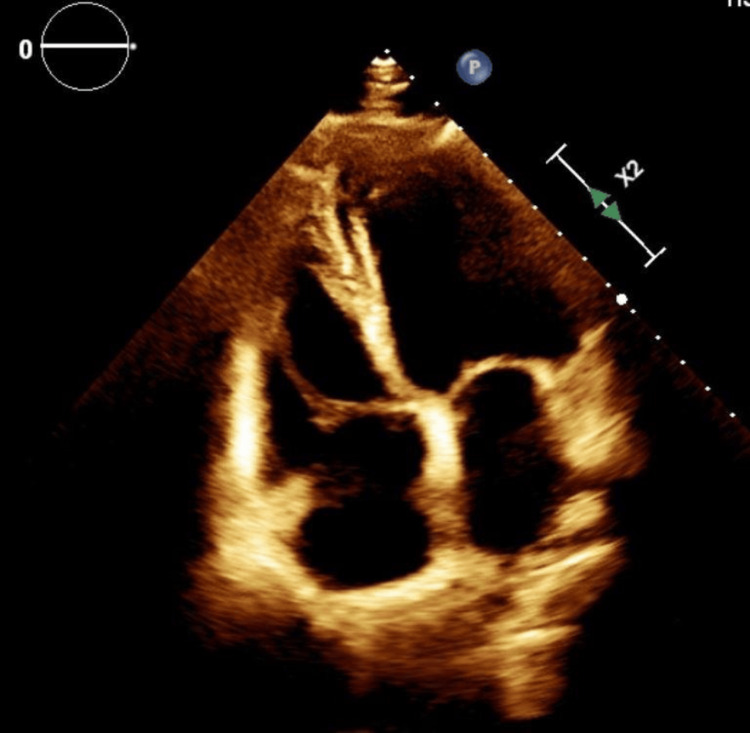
Follow-up TTE showing the resolution of mitral valve mass. TTE, transthoracic echocardiogram
